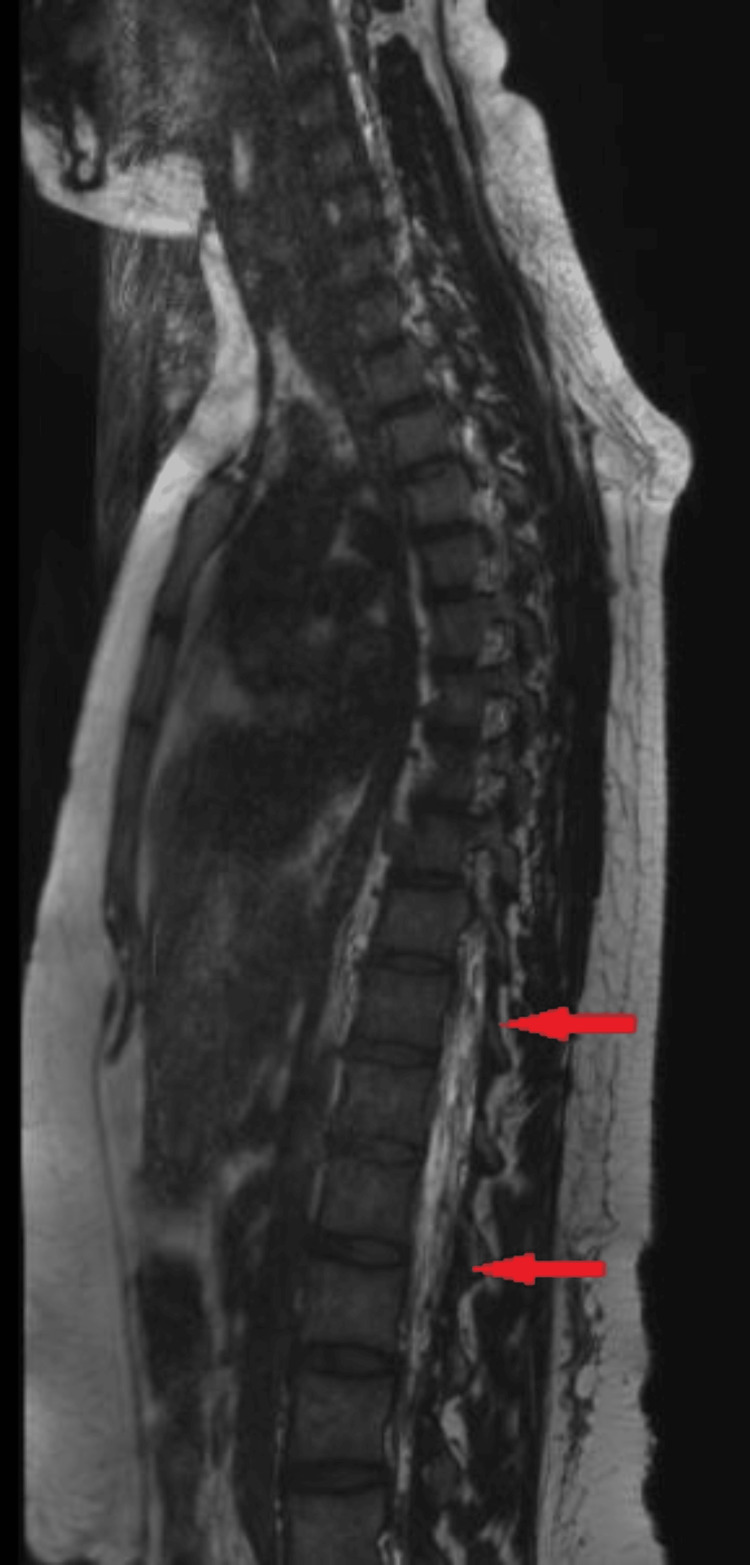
MRI results: diffuse extradural CSF accumulation (within the two arrows) within the thoracolumbar regions with evidence of intracranial hypotension
